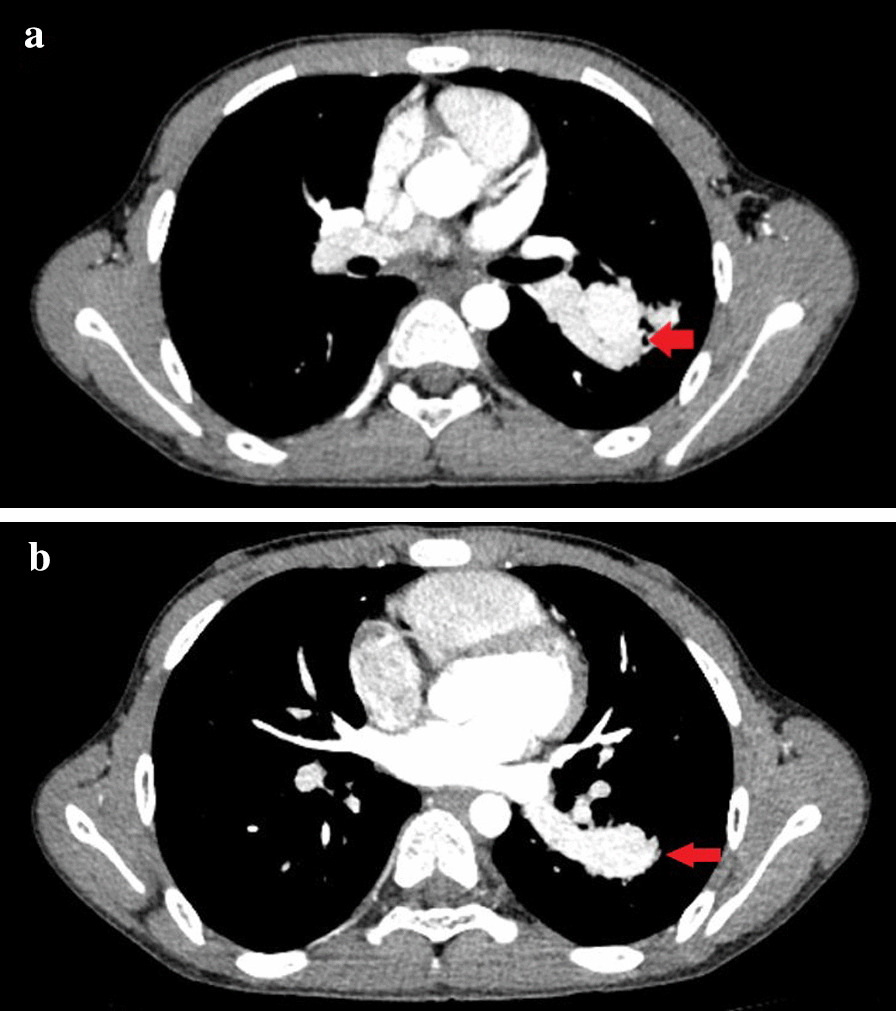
a, b CTA scan showed a large 3.8 × 3.2 cm lesion in the left lower lobe. (Mediastinal window)
